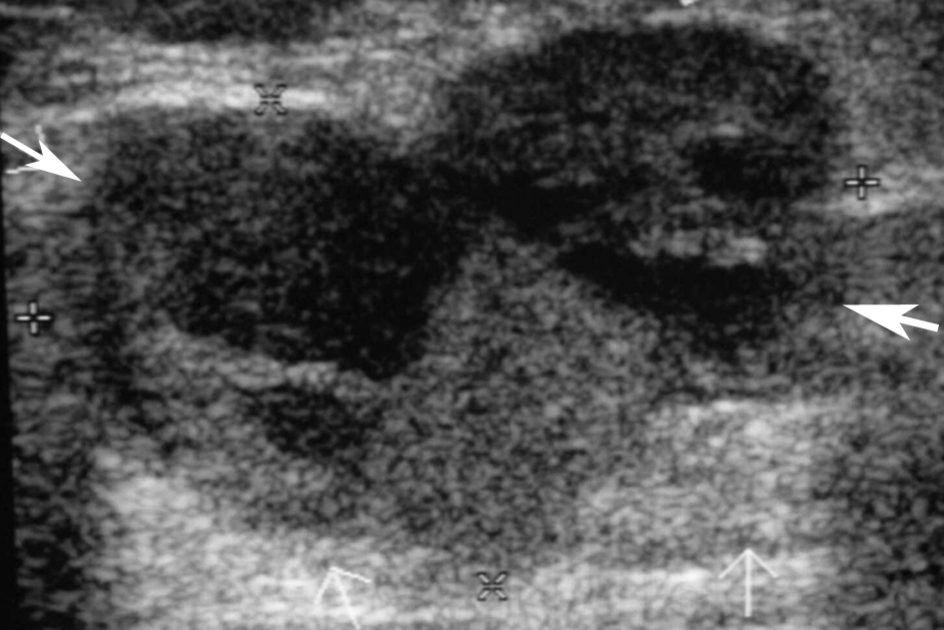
The corresponding breast ultrasound of the patient in Figure 1 shows a lobular mass at left lower breast, measuring about 3.1 × 2.1 cm in diameter with heterogeneous echogenicity (arrows).
